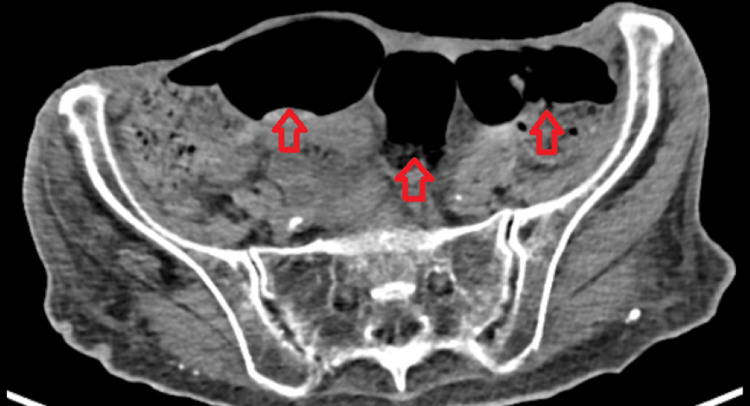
Abdominal CT scan Abdominal CT scan revealed largely dilated bowel loops (red arrows) with no signs of obstruction
